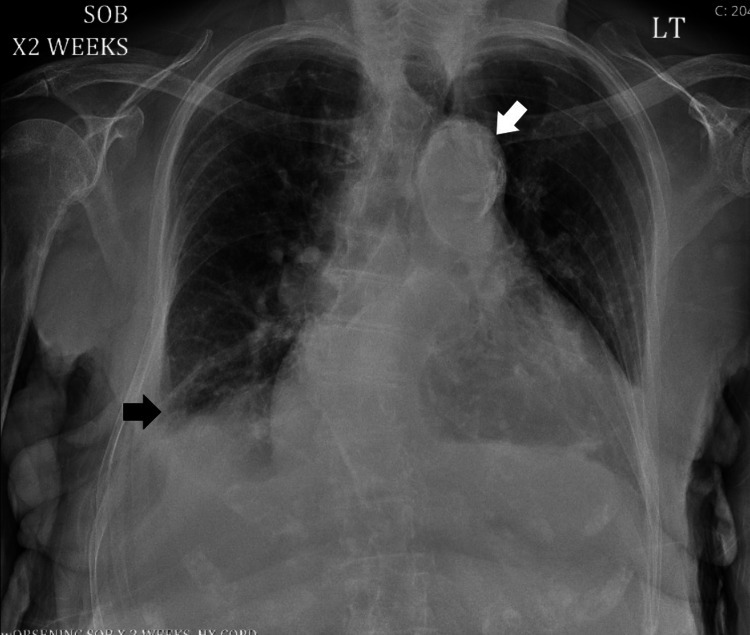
Chest radiograph showing a prominent and calcified aortic knob (white arrow). Additionally, a patchy right basilar opacity and a small right pleural effusion (black arrow) are seen.
